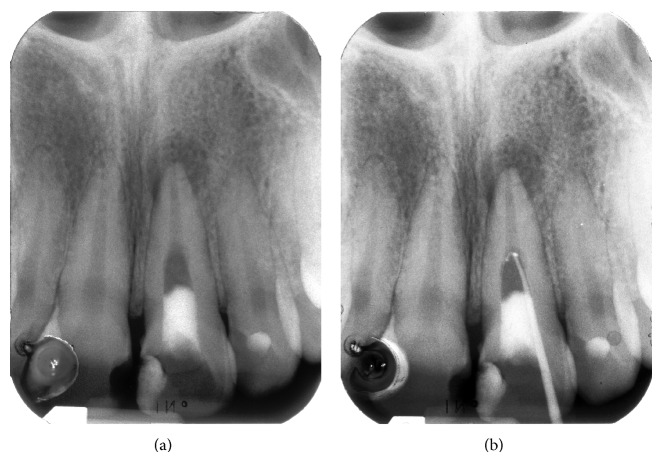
(a) Radiograph of tooth 21. (b) A gutta-percha point inserted in fistulous tract showing the midroot facial perforation.
